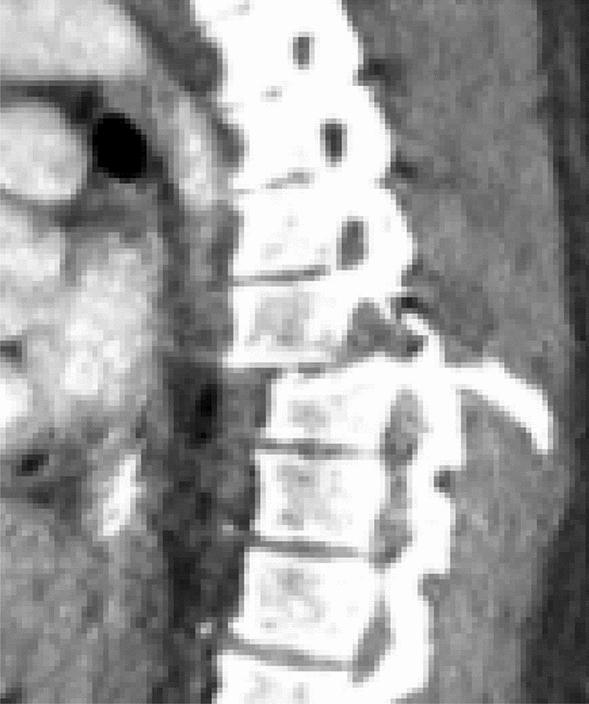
Rotational fracture-dislocation mechanism, CT scan, sagittal view
